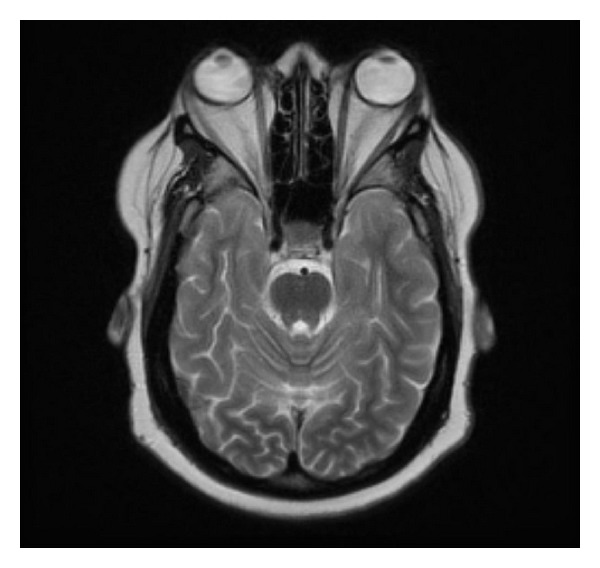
Bilateral exophthalmos as seen on MRI.
